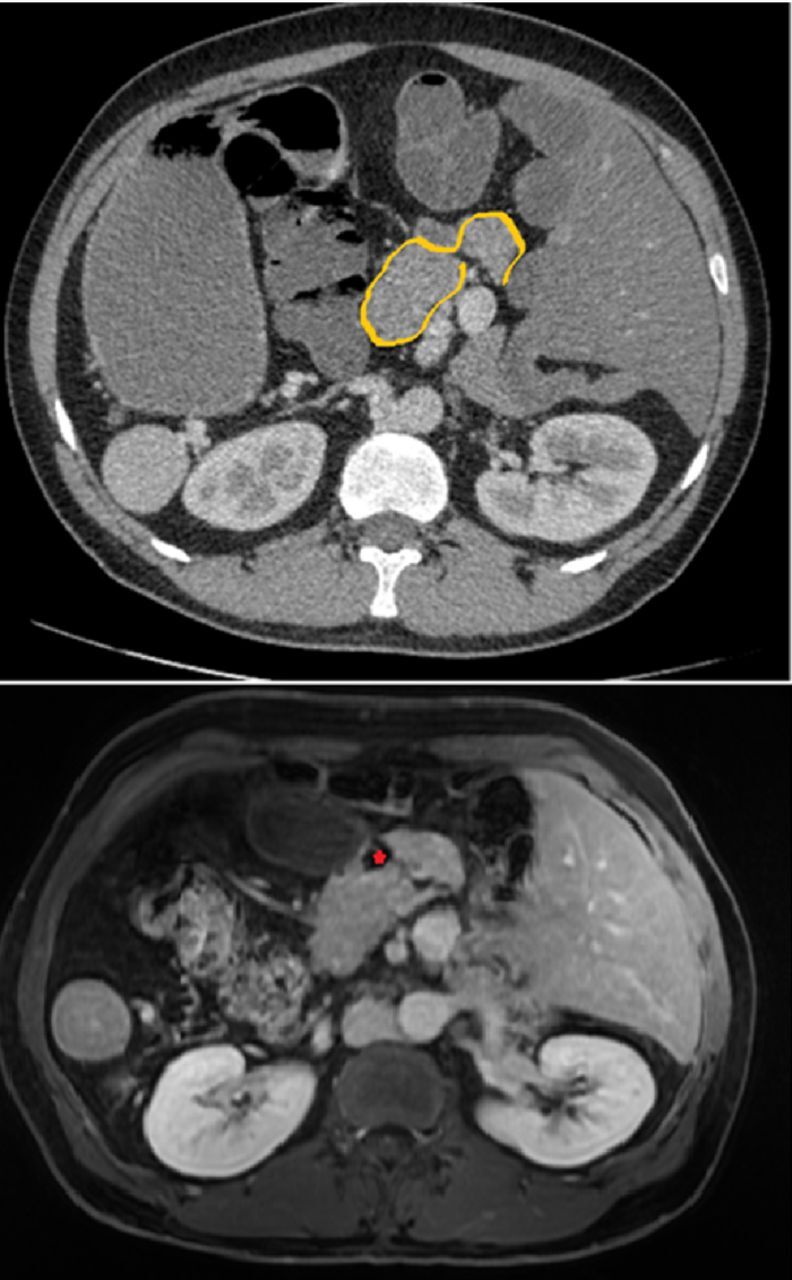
(Top) Fat-saturated axial T1-weighted computed tomography image shows the partial annular pancreas (pancreatic tissue outlined in yellow) with the duodenum located anteriorly. (Bottom) Contrast-enhanced axial fat-saturated computed tomography image shows the pancreatic tissue almost completely surrounding the second section of the duodenum (red star).
